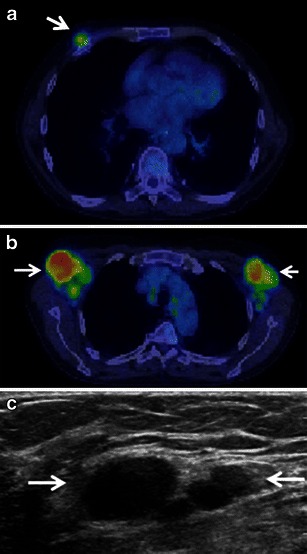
A 76-year-old man with known CLL presents with new palpable mass in the medial right breast which could not be included on a mammogram. Biopsy showed CLL. a Fused PET/CT image shows a hypermetabolic mass corresponding to the palpable mass in the medial right breast. b Fused PET/CT image demonstrates hypermetabolic axillary adenopathy (*arrows*). c Ultrasound shows a cluster of multiple hypoechoic masses with microlobulated margins without increased through transmission (*arrows*)
